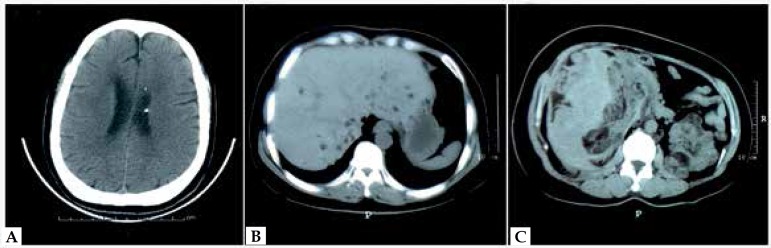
A: Brain CT shows two calcified nodules on the lateral
